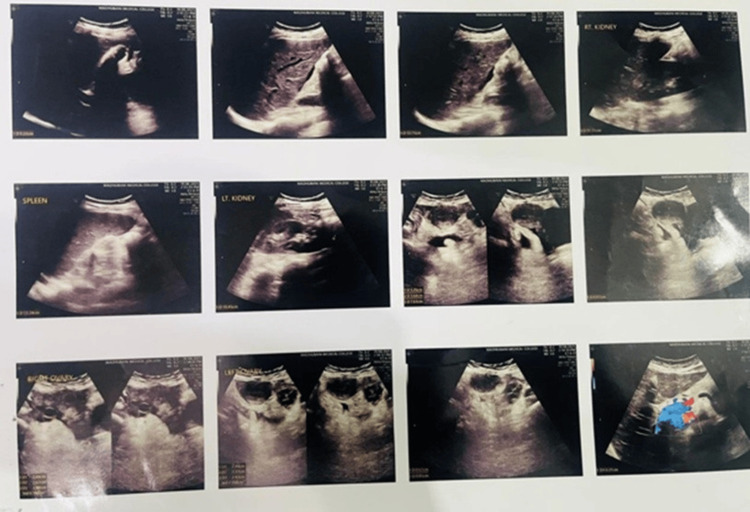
Ultrasonographic images showing gallbladder calculi with posterior acoustic shadowing and mild pericholecystic fluid
